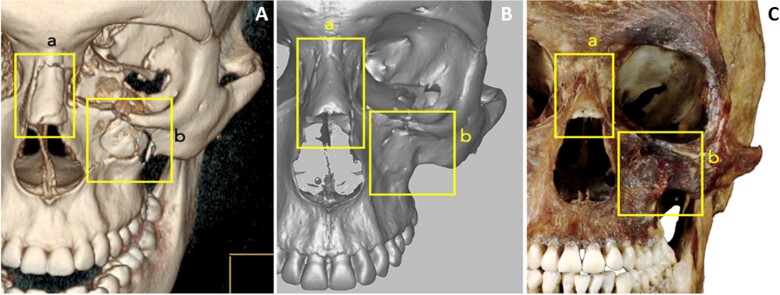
Antemortem CT scan reconstruction (A), postmortem 3D surface scan (B), and postmortem photography (C) of the left craniofacial structures. Highlighted in the yellow boxes are a nasal bone fracture (a) and a left maxillary fracture (b). The fractures appear unhealed in the antemortem CT scan (A) and healed in the postmortem images (B, C).
